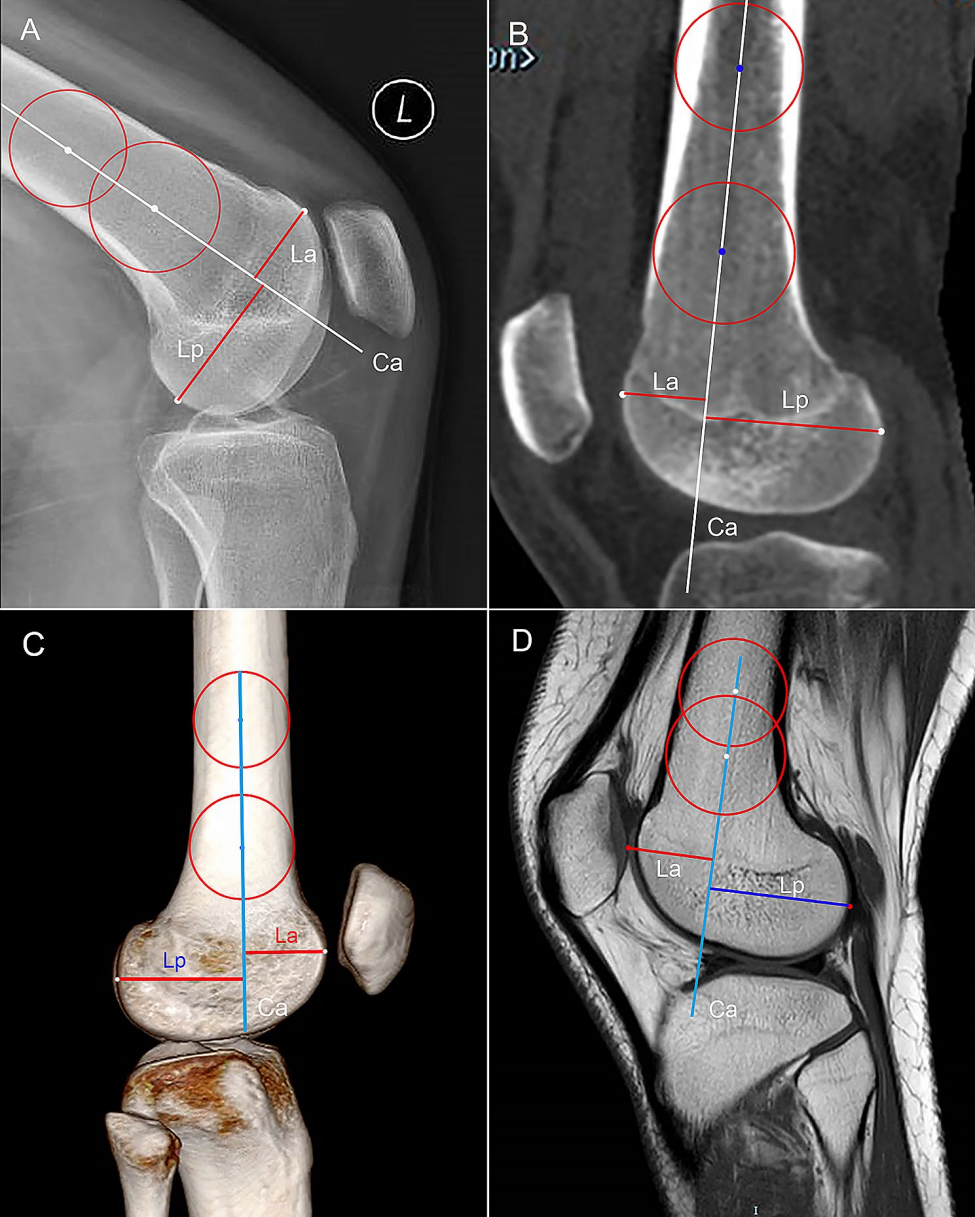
The measurement of lateral femoral condyle ratio (LFCI). (A) Lateral view of conventional radiograph is selected. Two circles are drawn to tangent with the anterior and posterior femur cortex, and the central longitudinal axis (Ca) passing through the centers of the two circles is identified. The vertical length between the most posterior or anterior portion of lateral femoral condyle and the Ca (Lp and La, respectively) is measured to calculate LFCI: Lp/La. (B) midsagittal CT slice is selected. (C) 3D–CT is reconstructed and standard lateral view is selected. (D) Midsagittal MRI slice is selected, and the Lp and La is the vertical distance between the cartilaginous margin (red dots) of femoral condyles and the Ca, respectively
